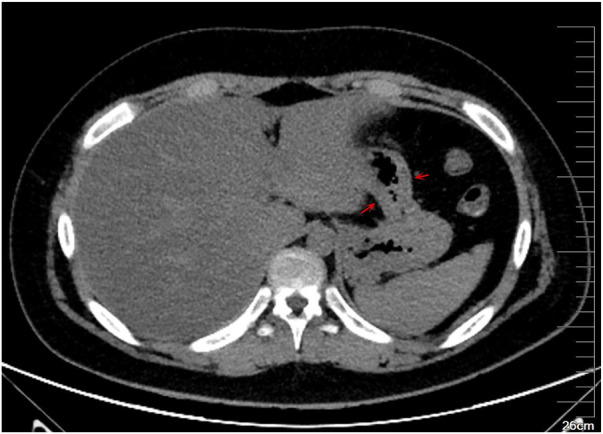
The abdominal CT image shows that the gastric wall has thickened.
